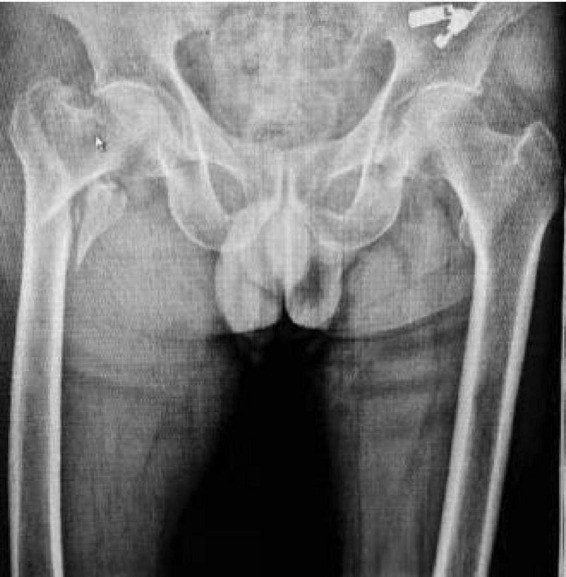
Preoperative anteroposterior radiograph of both hip joints showing unstable right intertrochanteric femoral fracture in a 78-year-old male patient. The fracture pattern demonstrates characteristic features of instability requiring surgical intervention.
